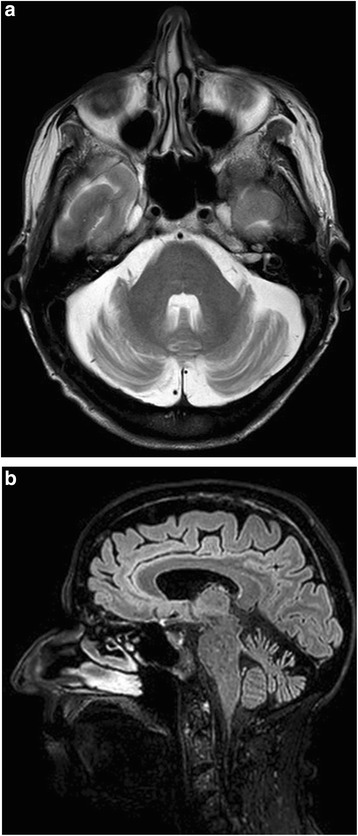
a Axial MRI T2 image showing atrophy of the cerebellar hemispheres (grade 1). b Sagittal MRI image, showing vermian grade 2 atrophy
